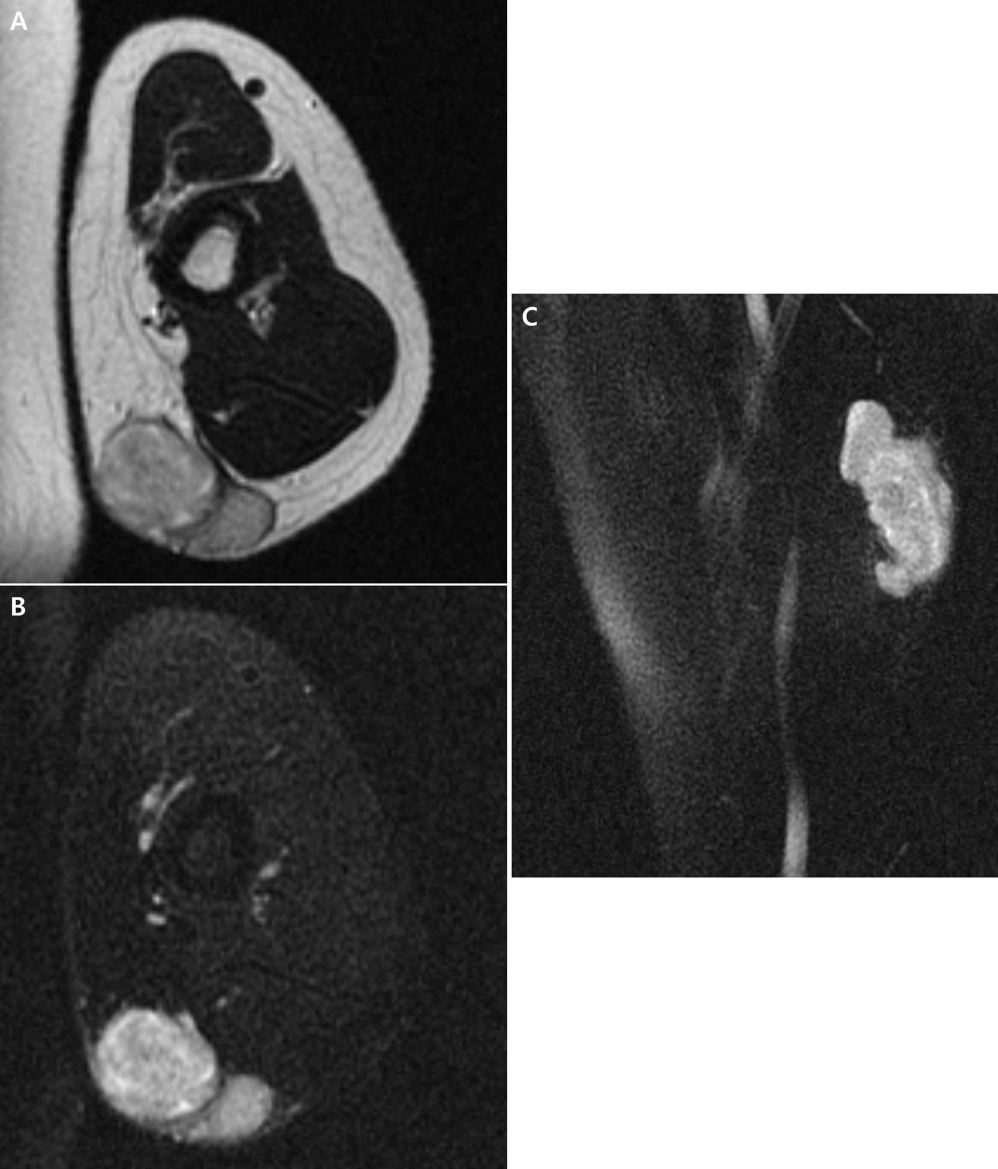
Magnetic resonance images of a 53-year-old woman with myxofibrosarcoma before unplanned excision. A Axial T2-, B axial fat-suppressed T2-, and C sagittal fat-suppressed T2-weighted images of left upper arm demonstrate a 4.4 cm-sized soft tissue mass in the subcutaneous fat layer with lobulated contour.
